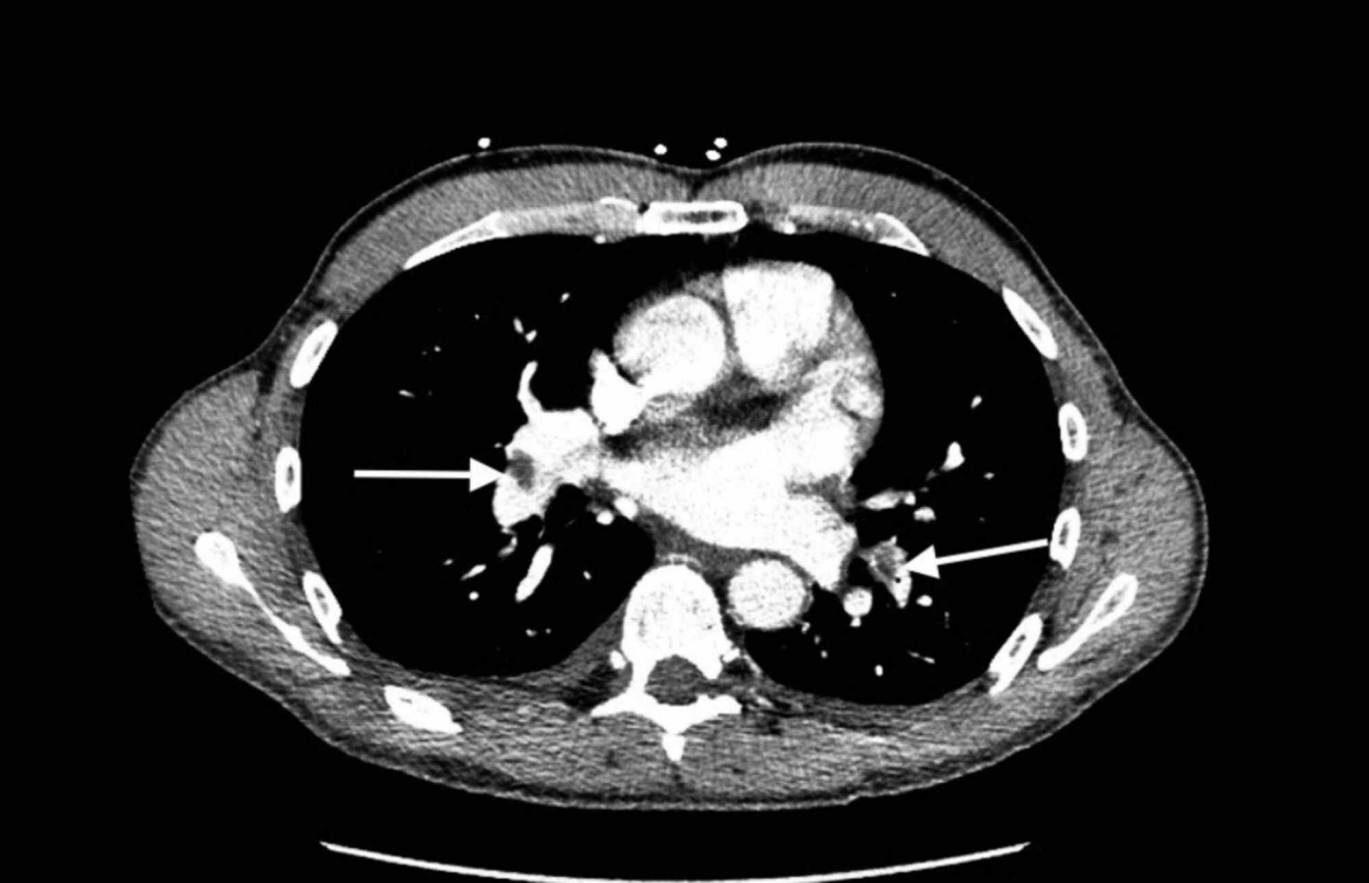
Bilateral pulmonary embolism (PE) CT scan of the chest with contrast revealed bilateral segmental and subsegmental PE within the bilateral lower lobes and right middle lobe.
